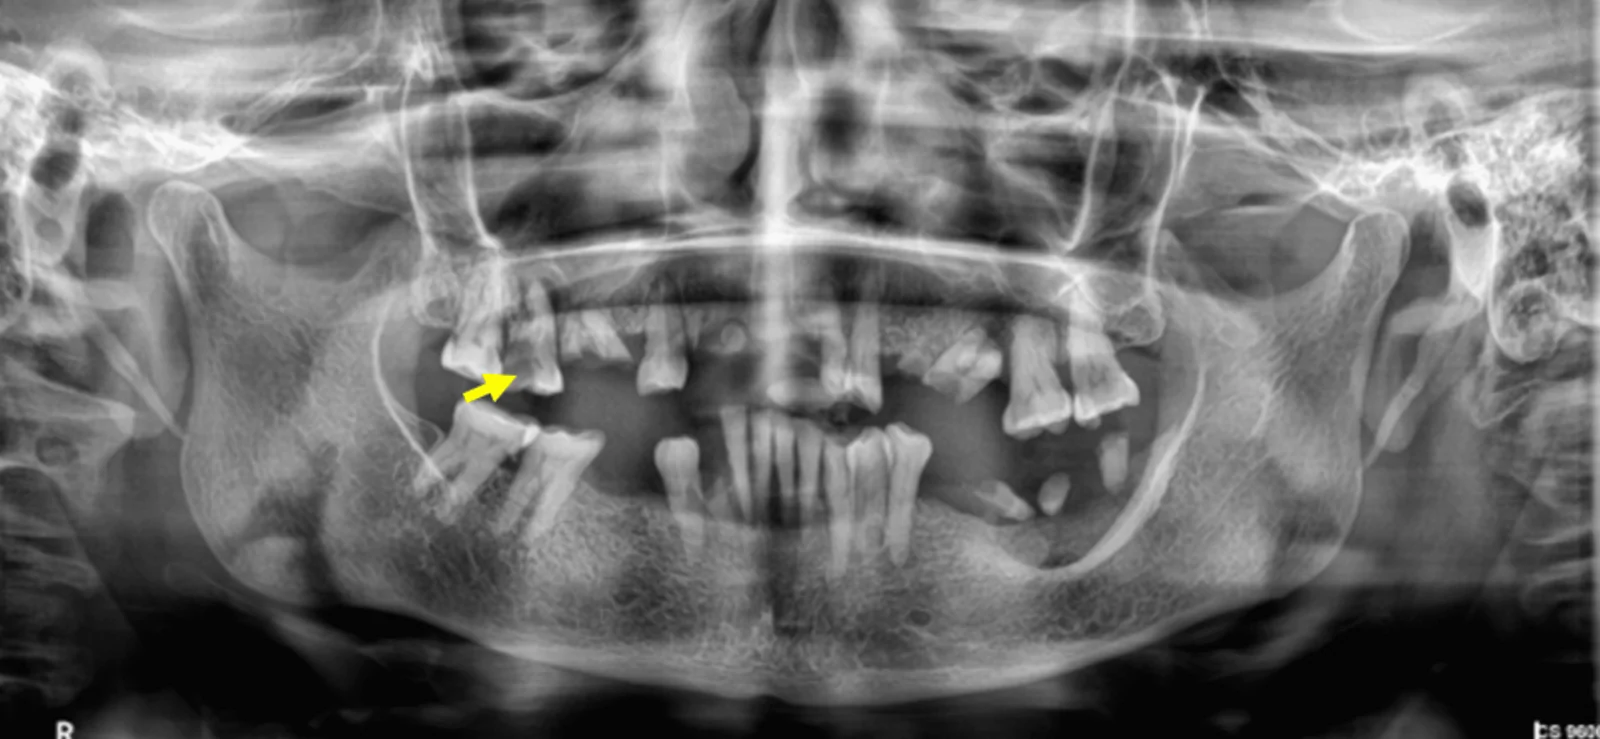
Case one - orthopantomography revealed radiolucency in the distoproximal crown portion of right maxillary second molar tooth, suggestive of distoproximal caries (yellow arrow indicates the affected carious tooth).
